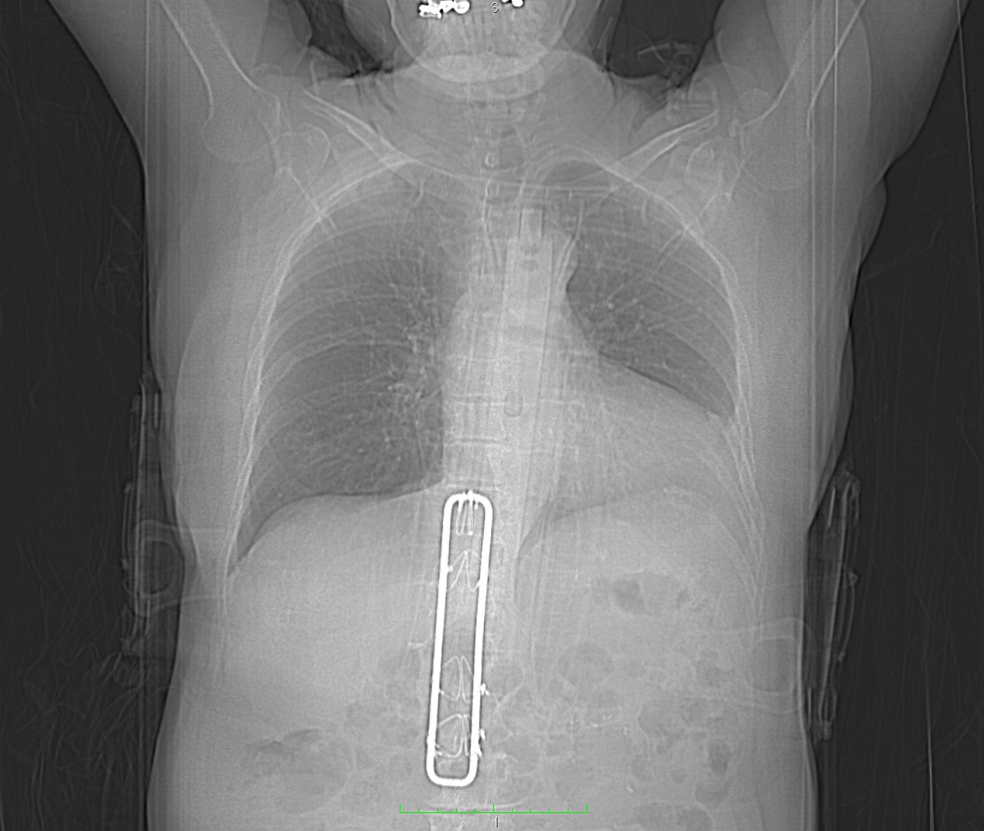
Preoperative X-ray Preoperative X-ray identifying Hartshill rectangle and sublaminar wires.
